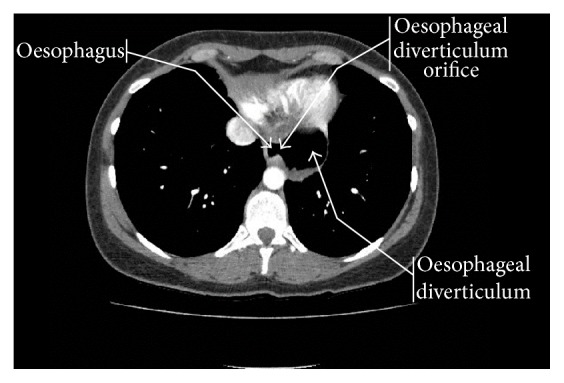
Epiphrenic oesophageal diverticulum containing food residue. CT chest with IV contrast.
